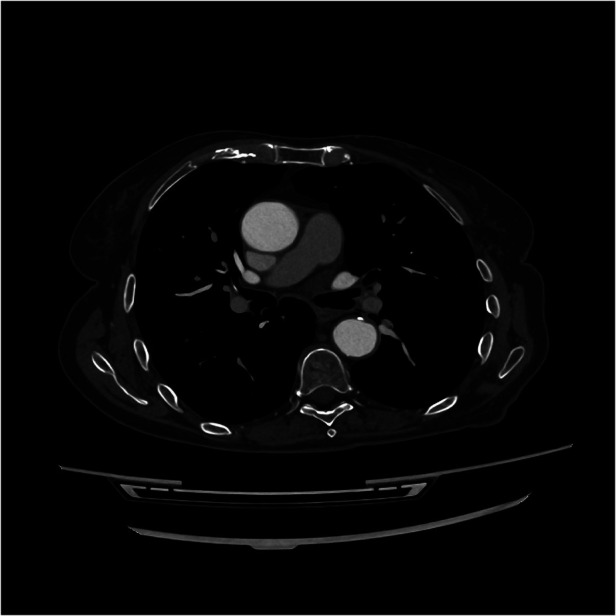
Transversal CT-scan plane showing the resolution of the 8 mm thrombus in the ascending aorta.
